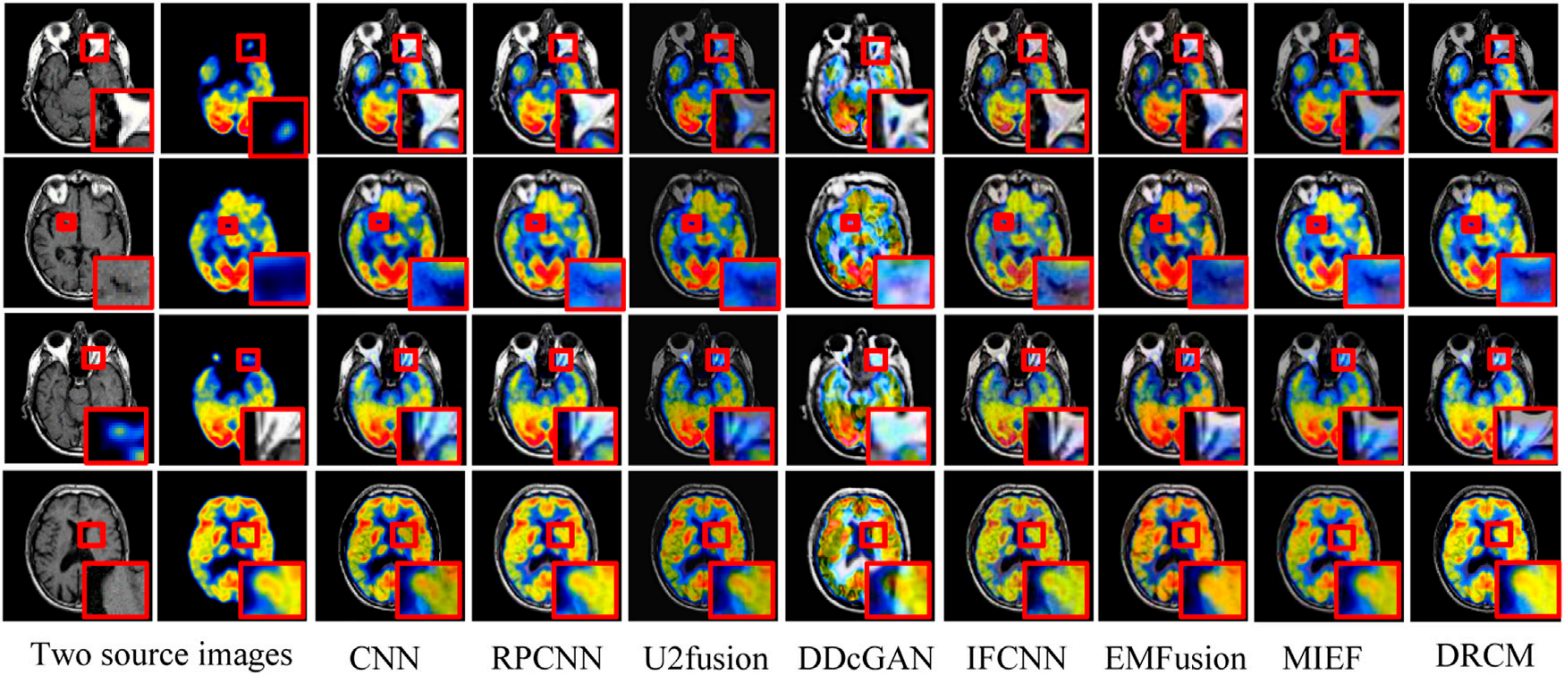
PET–MRI fusion images under different comparison methods. Two source images are reproduced with permission from http://www.med.harvard.edu/AANLIB/home.html.
